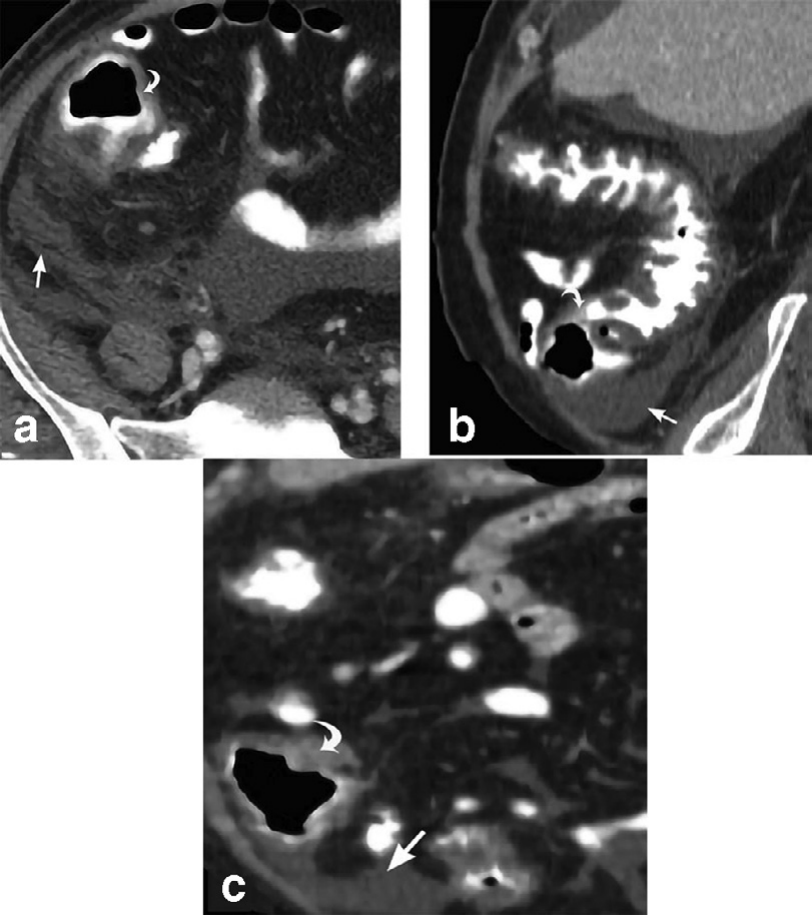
Pseudomembranous colitis. 54-year-old male presents with diarrhea for 3 days. Diffuse colonic wall thickening involving the cecum (curved white arrows) is depicted on axial CT (a) and sagittal MPR CT images (b, c). There is surrounding inflammatory soft tissue stranding and free fluid (straight white arrows). Subsequent stool assay reveals that the patient was positive for the *C. difficile* toxin. Sigmoid biopsy reveals colonic mucosa with superficial necrosis and fibrinopurulent exudate. MPR, multiplanar reconstruction.
